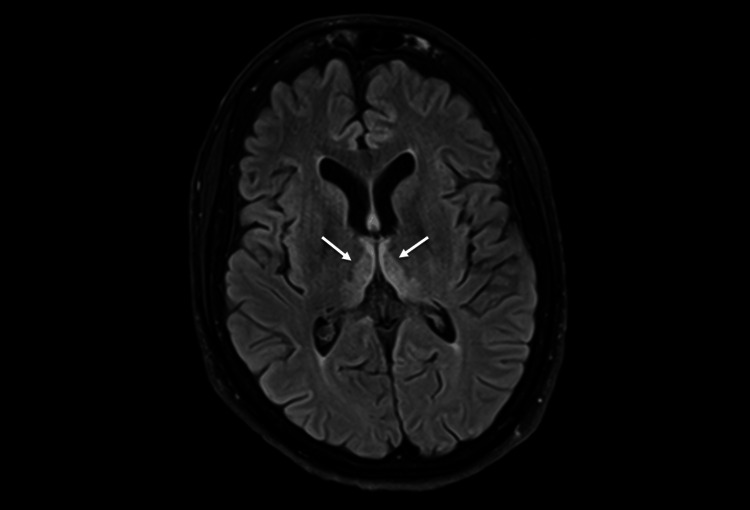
Axial T2 FLAIR, thalamic dorsomedial bilateral symmetric hyperintensity FLAIR: fluid-attenuated inversion recovery
